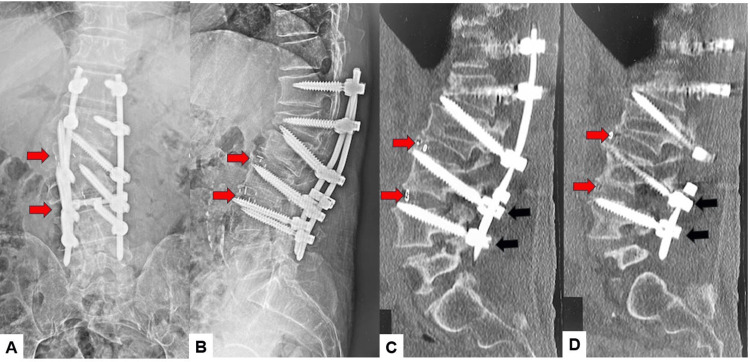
Final follow-up images A: Anteroposterior lumbar radiogram, B: Lateral lumbar radiogram, C: Left parasagittal lumbar reconstruction CT, and D: Right parasagittal lumbar reconstruction CT. Red arrows indicate OLIF cages. Block arrows showed transdiscal screws. OLIF: Oblique lateral interbody fusion
